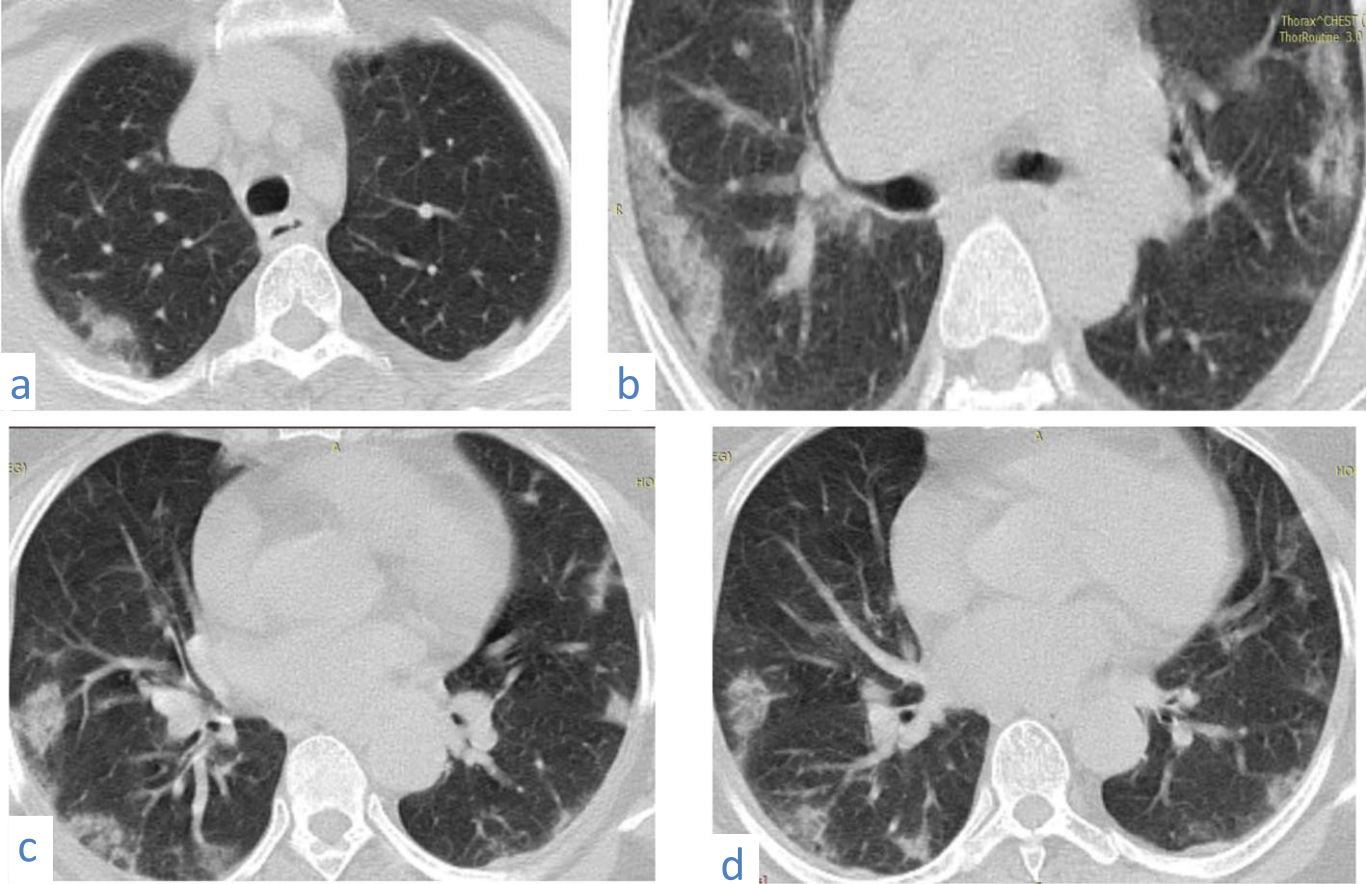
A 57-year-old male patient with COVID-19, CT chest axial images (a–d) show bilateral predominantly subpleural consolidation with tiny lucencies within “vacuolar sign”
